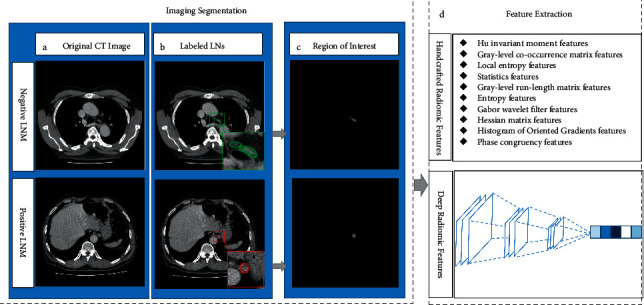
The extraction of radiomic features. (a) Original CT images with and without lymph node metastasis (LNM). (b) Labeled lymph nodes (LNs), negative LNM in the green box, positive LNM in the red box. (c) Region of interest (ROI) with and without LNM. (d) Feature extraction of handcrafted radiomic features and deep radiomic features.
